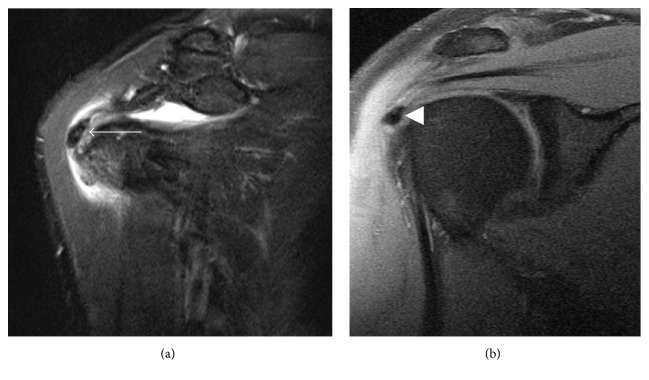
MRI appearance of calcific tendinopathy. (a) The acute, symptomatic phase of calcific tendinopathy is often associated with soft tissue edema surrounding the tendon calcifications (arrow). (b) In the chronic phase of calcific tendinopathy, calcifications are typically more well defined and homogeneously low in signal intensity without surrounding edema (arrowhead).
